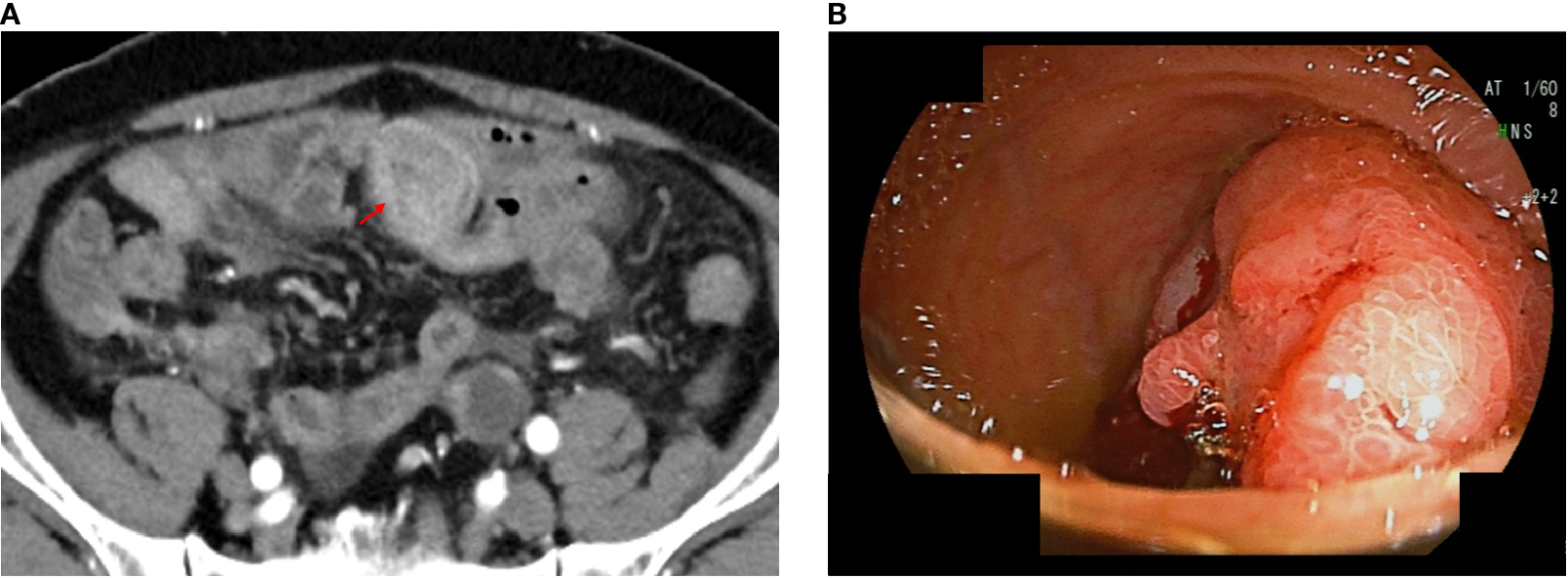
Computed tomography (CT) and endoscopic images of unresectable Meckel’s diverticulum cancer. CT images performed at our hospital; the arrow indicates an enhanced mass lesion in the ileum (a). Double-balloon endoscopic finding of Meckel’s diverticulum cancer (b).
